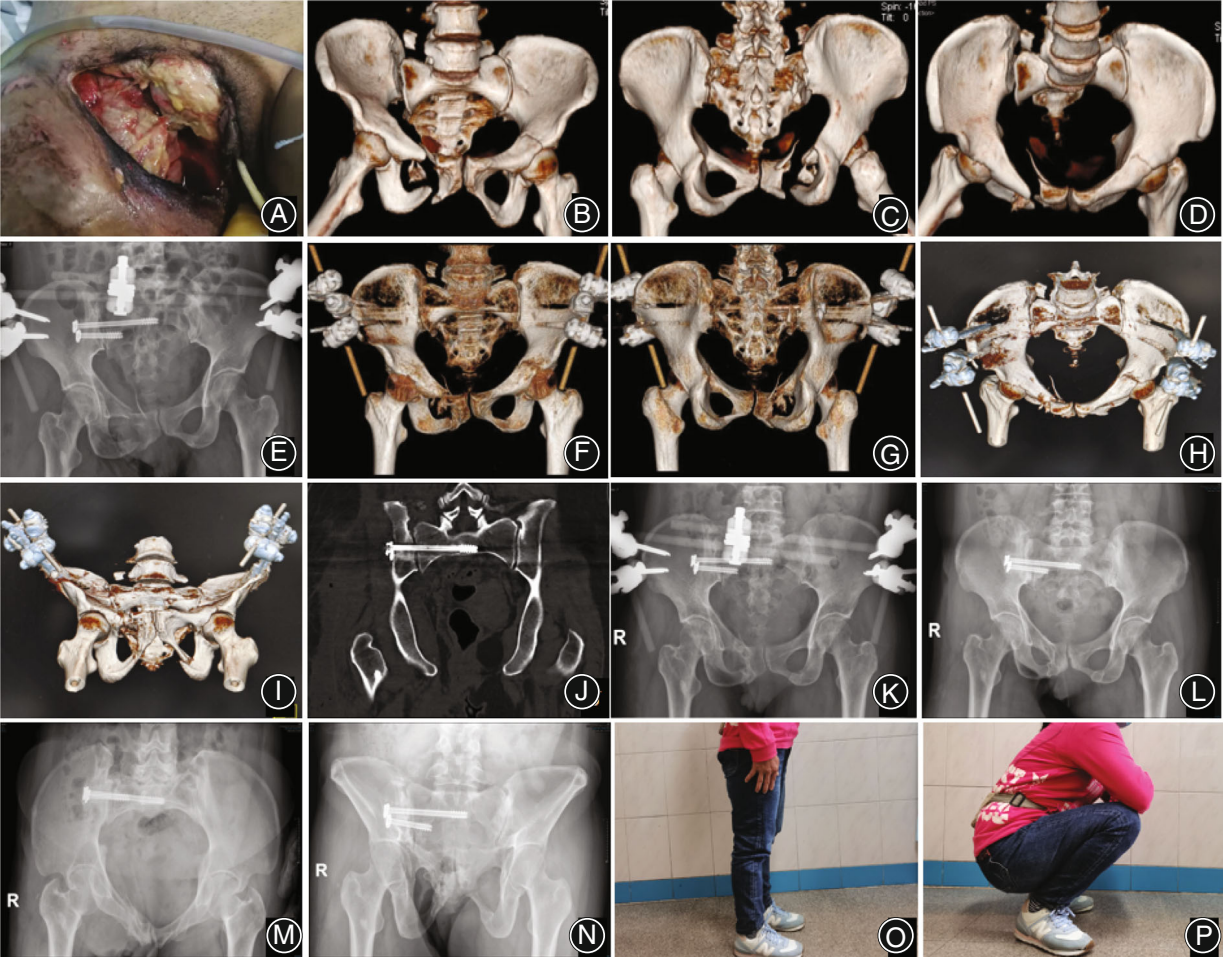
A 43‐year‐old woman with pelvic ring injures (Case 1) caused by a traffic accident. (A) Open injury of right perineum. (B, C, D) Preoperative three‐dimensional computed tomography image showing sacroiliac joint dislocation and bilateral pubic ramus fractures. (E–J) Two days postoperative plain X‐ray film and three‐dimensional computed tomography images showed satisfactory reduction after countertraction closed reduction technique and the fixator was in the right position. (K–N) Three‐month postoperative plain X‐ray radiographs showing healed fractures and the external fixator was removed. (O, P) Follow‐up 3 years after surgery showed good function.
